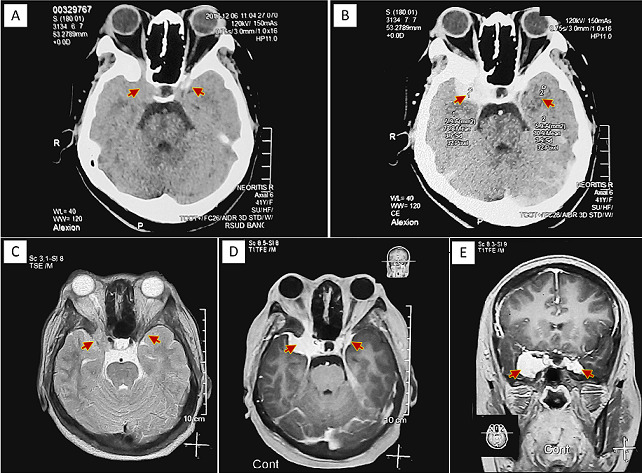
right sphenoid wing meningioma showed (red arrows) by homogenous enhancing solid mass in the right and left suprasellar regions, with hyperostosis; depicted in the axial slice of the; A) non-contrast CT-scan; B) contrast CT-scan; C) non-contrast MRI; D) contrast MRI, and coronal slice of; E) contrast MRI
